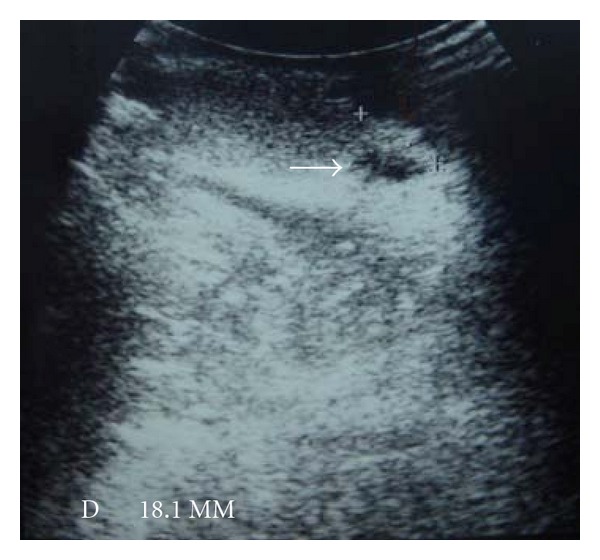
Scrotal ultrasound showing a focal cystic paratesticular mass with compressed testicular parenchyma (arrowhead).
